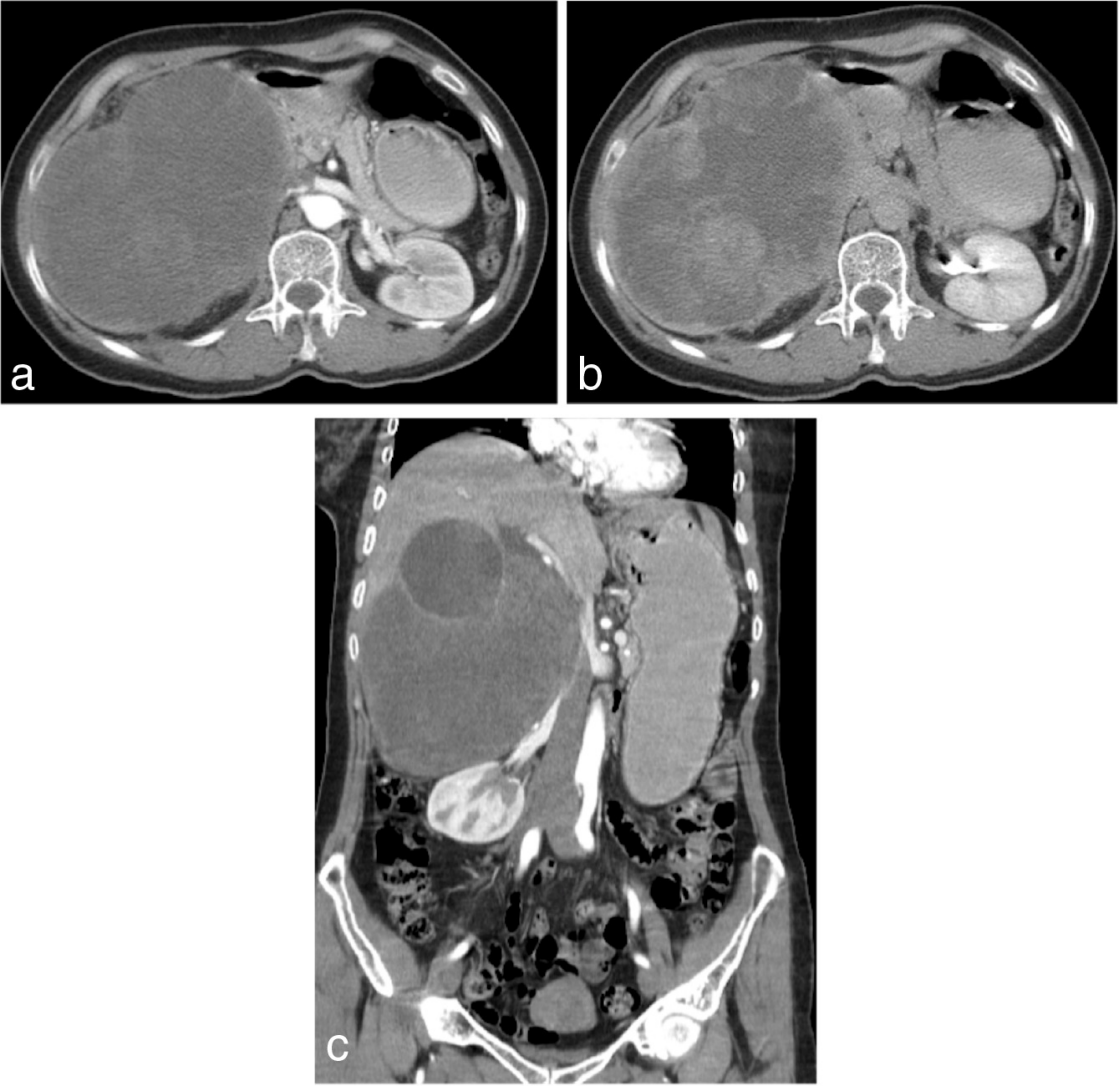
MDCT images obtained at admission. Transverse iodinated contrast-enhanced MDCT at the level of the left renal hilum in the arterial (a) and interstitial (b) phases showed a heterogeneous, expansile low soft tissue density mass abutting the inferior surface of the gallbladder and the right hepatic lobe, and compressing the hepatic hilum. It is hypovascular (a) with progressive enhancement on later phases (b). Coronal reformatted contrast-enhanced MDCT image of the abdomen and pelvis demonstrates an enlarged and distended gallbladder with heterogeneous filling (c). MDCT, multidetector CT.
